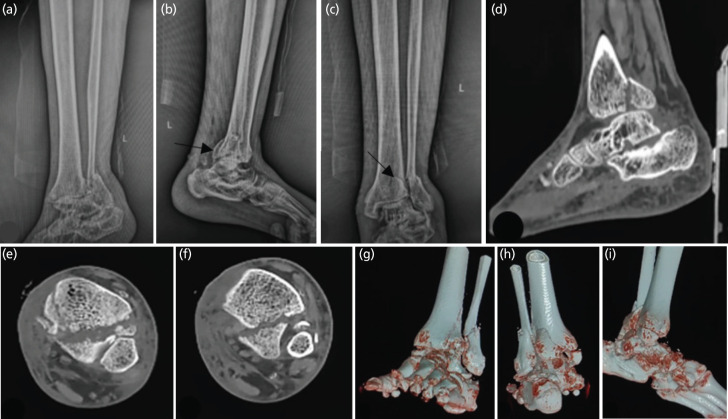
Pre-operative radiographs and CT of a 56-year-old male patient; (a) shows anteroposterior view radiograph of the ankle, we can identify the fractured lateral and medial malleolus; (b) shows lateral view radiograph, here we can identify the posterior malleolar fracture fragment (arrow); (c) shows a mortise view radiograph of the ankle in which the fracture pattern is better appreciated and the posterior malleolar fracture fragment appears to be posterolaterally located (arrow); (d) shows the sagittal cut CT scan 2D image of the ankle; (e) shows the axial cut CT scan 2D image of the ankle here we can identify that the posterior malleolar fracture fragment involves the whole of the posterior malleolus distally; (f) shows another axial cut CT scan 2D image of the ankle which is a few cms proximal to the previous one; (g, h, i) show 3D reconstructed images of the ankle showing a posterolateral posterior malleolar fracture fragment along with a transverse medial malleolar fracture and an oblique lateral malleolar fracture.
